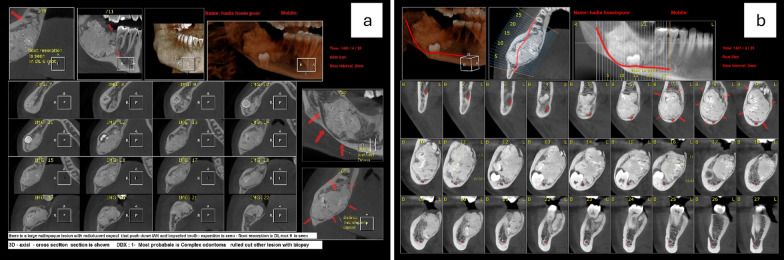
Preoperative cone-beam computed tomography. A Axial, B sagittal
